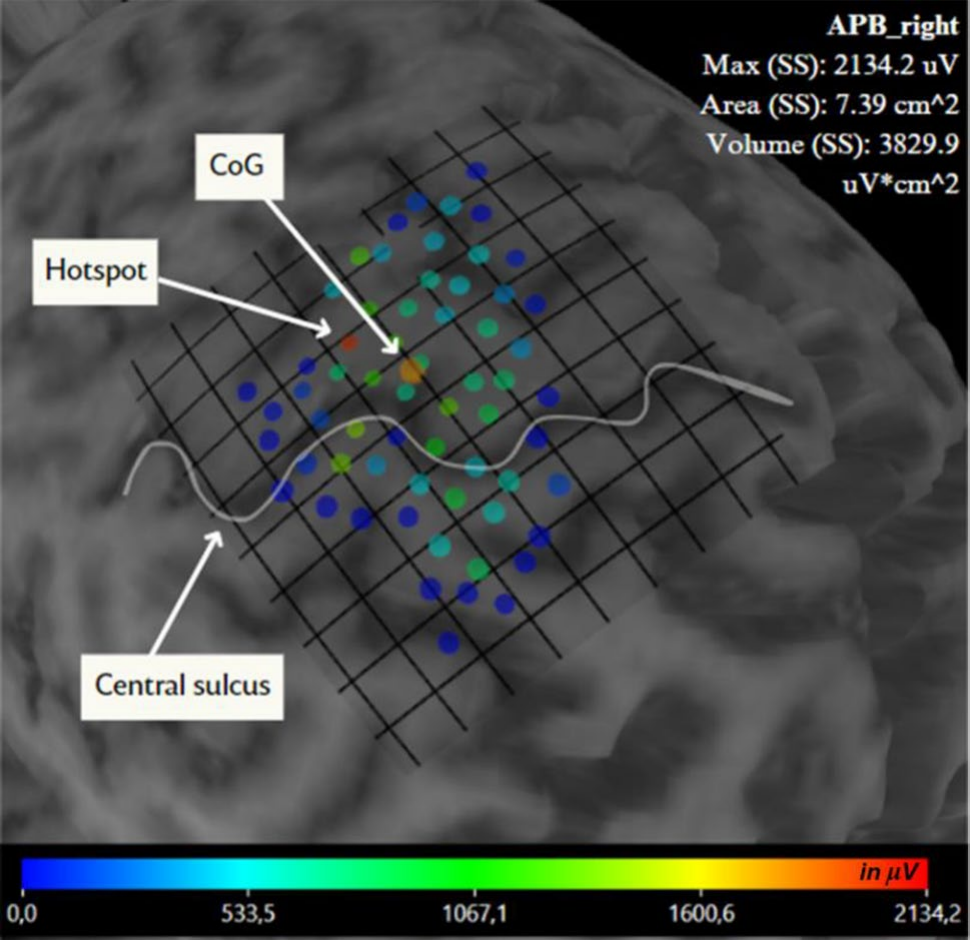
The MCR of the APB in a representative subject is visualized in TMSmap software (Novikov et al. 2018). Colored dots represent the stimulation points. The color reflects the peak-peak APB MEP amplitude in μV (see the color bar), so the red dot represents the hotspot. The big orange dot represents the CoG. The white line marks the central sulcus on the individual MRI. The overlaid grid has 5 × 5 $${mm}^{2}$$ square size. The APB MCR area and volume are shown in the upper right corner
